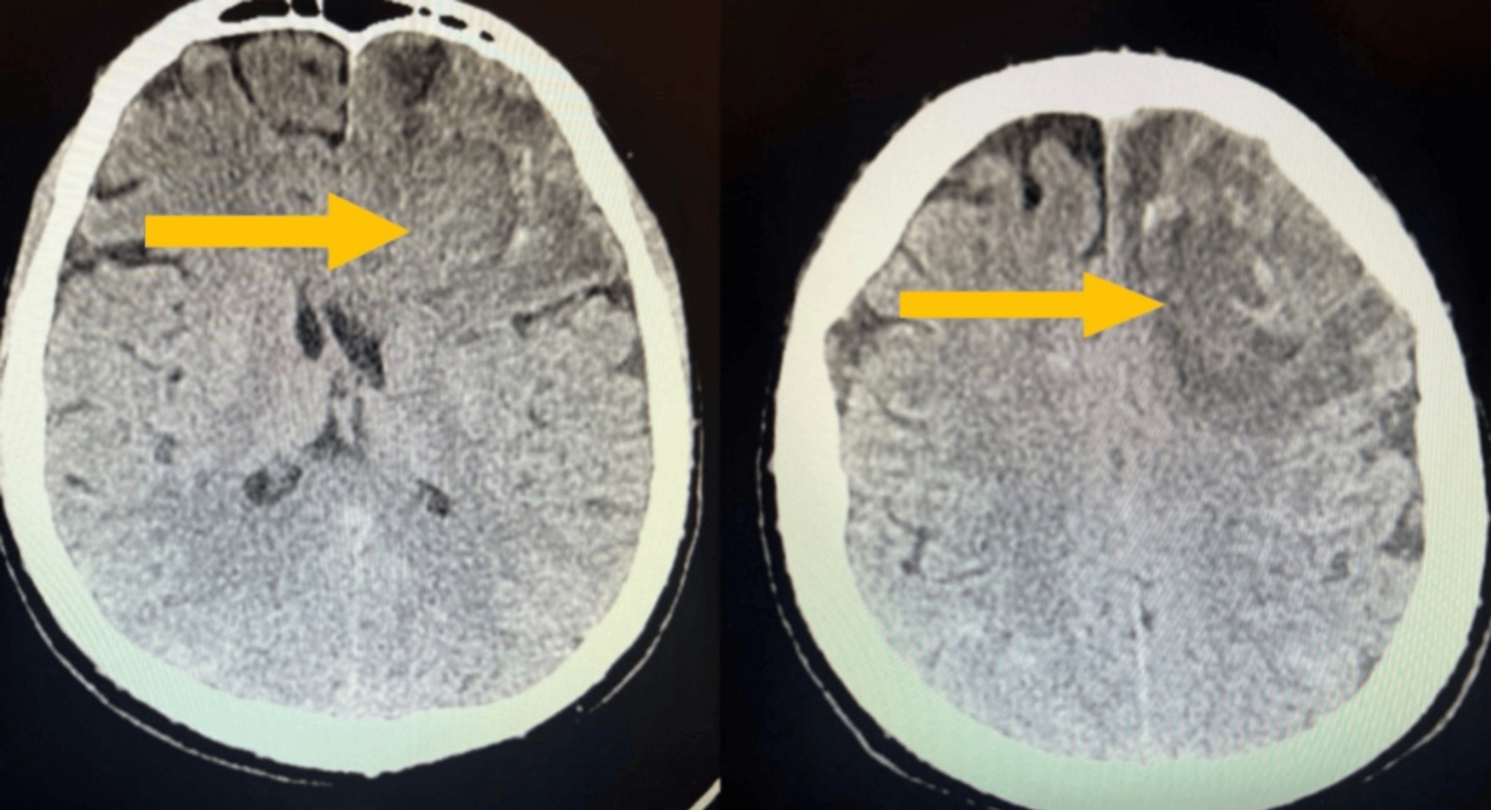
Follow-up CT scan of the head on day 15 of treatment Follow-up CT on day 15 showing marked hemolysis of the left frontal hematoma with surrounding edema and residual mild subarachnoid hemorrhage in the posterior parietal and occipital sulci.
